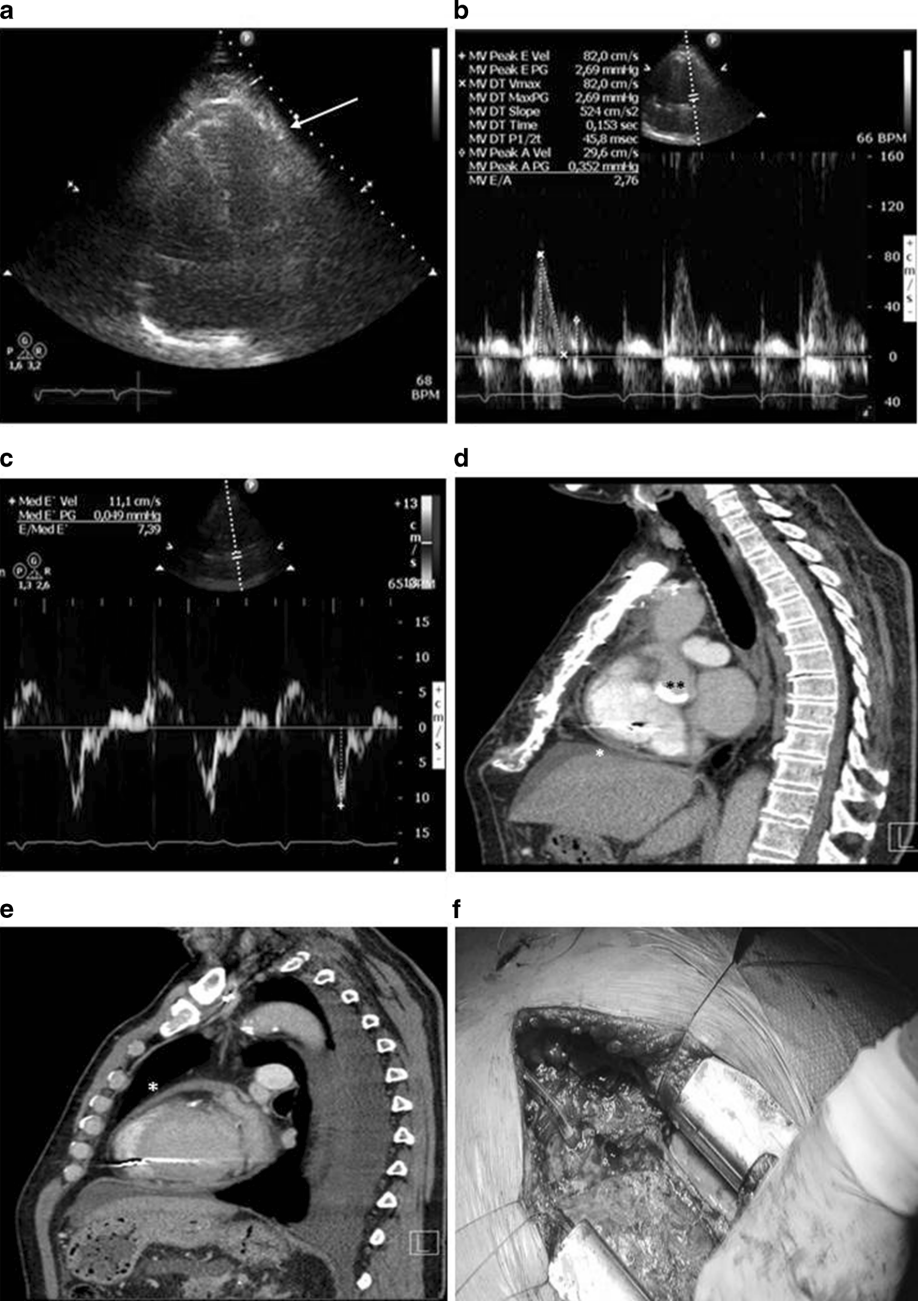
a. Transthoracic echocardiographic image of apical four-chamber view, demonstrating normal LV function with an undefined echodense layer or mass at LV apex b. Transthoracic echocardiographic image of transmitral inflow pattern using pulsed wave Doppler imaging c. Transthoracic echocardiographic image of mitral annual velocity using tissue Doppler imaging of septal mitral valve annulus movement demonstrating normal LV fillings pressure (E/E’ ratio of 7.4) d. CT image of the pericardium showing thickened pericardium without calcifications by *single asterisk* and the On-X aortic valve by *double asterisk*
